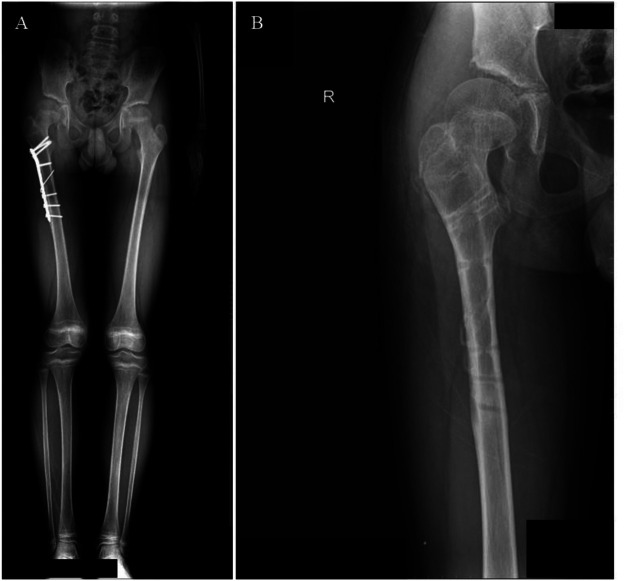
A full-length radiograph of both lower extremities, showed good healing of the right femoral shaft fracture at one-year follow-up, with the femoral head within the acetabulum and equal leg length; B demonstrated that after removal of the femoral shaft internal fixation, the right femoral head remains well-positioned with preserved morphology and no signs of AVN.
